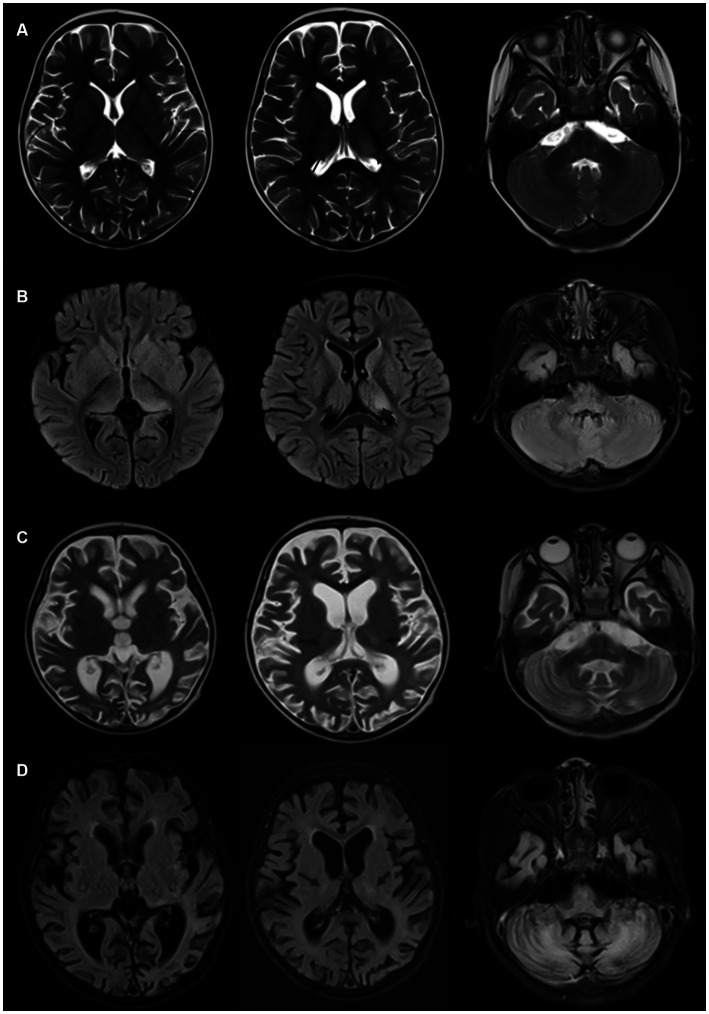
(A,B) Brain MRI of Patient 2, acquired one week after onset, demonstrating T2 and FLAIR hyperintense signals in the basal ganglia, dorsal thalamus, and bilateral cerebellar hemispheres. (C,D) Brain MRI of Patient 2, obtained one year after onset, showing a reduction in the original lesions in the basal ganglia and dorsal thalamus, along with marked global cerebral atrophy and significant ventricular dilation.
